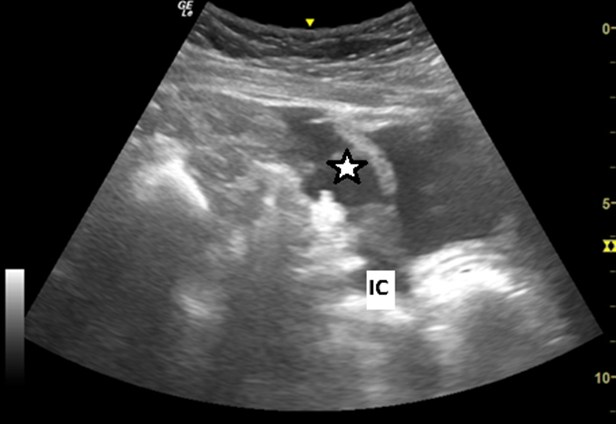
Point of care ultrasound (POCUS) image of the right pelvis and upper inguinal region in the longitudinal/oblique plane using the curvilinear 5-12 MHz probe. This demonstrates free fluid (*) in the pelvis communicating with the right inguinal canal (IC)
